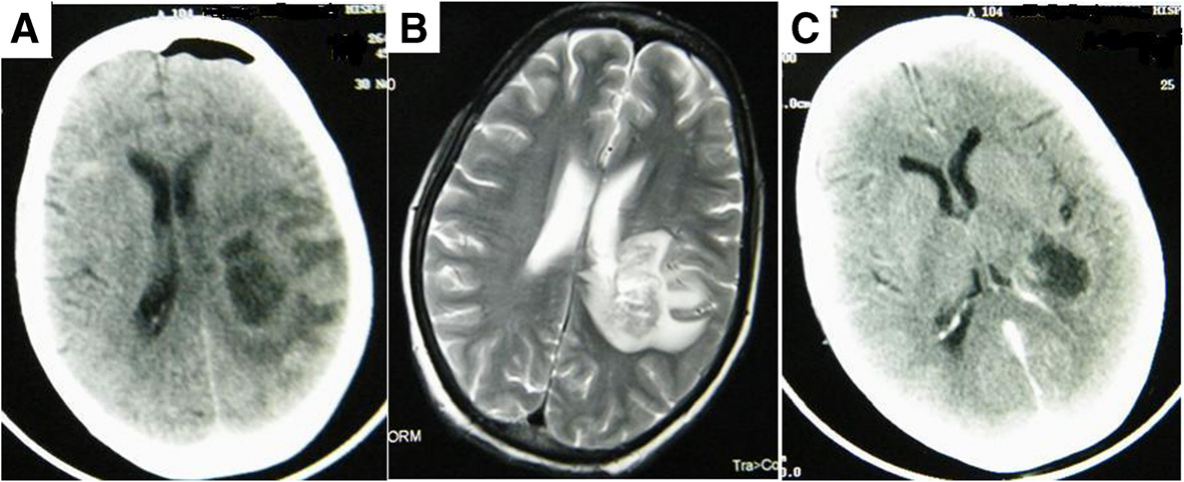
Radiology of head. (A) NCCT head showing a large hyperdense lesion in the right temporo-parietal region with peri-lesional edema, which is confirmed on MRI (B). Significant reduction in size of the lesion after drainage and antifungal therapy on repeat NCCT head (C).
